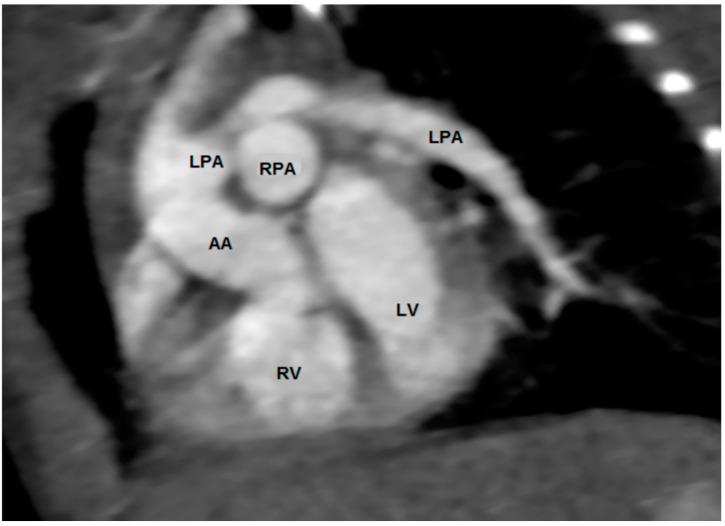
Computed tomography in the coronal plane. The left pulmonary artery arises from the ascending aorta and courses superiorly to cross the origin of the right pulmonary artery.
